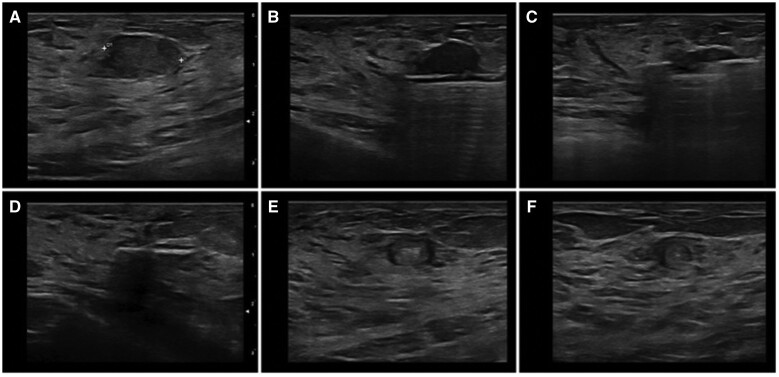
Step-by-step ultrasound-guided vacuum-assisted excision of a fibroadenoma. (A) solid hypoechoic lesion, with regular shape and a circumscribed margin (B, C) US guided 8G vacuum-assisted excised (D) lesion has been completed removed and metallic marker clip release (E) axial follow-up scan at 12 months (F) longitudinal follow-up scan at 12 months.^18^
